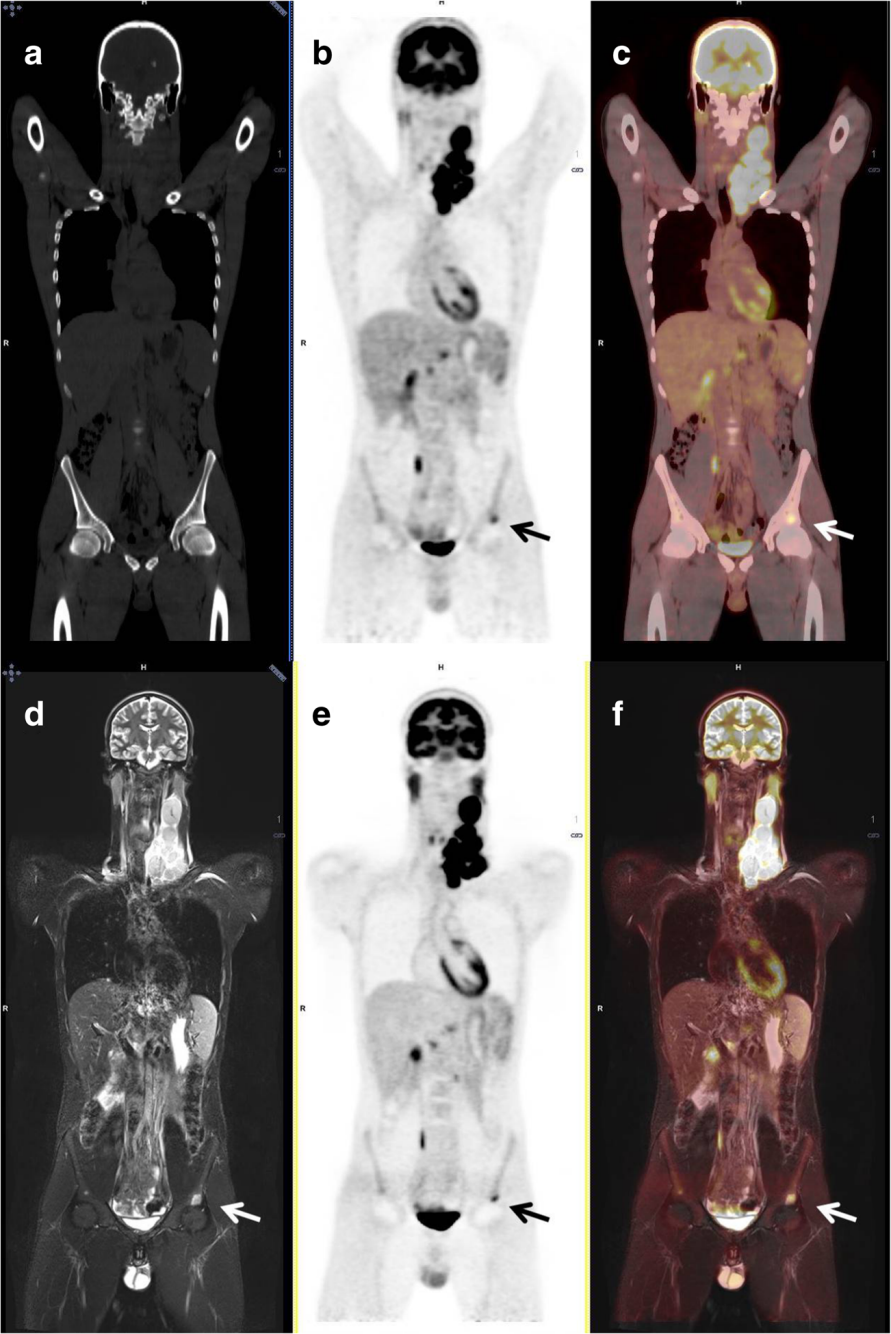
Coronal CT (a), coronal PET from PET/CT (b), fused coronal PET/CT (c), coronal STIR (d), coronal PET from PET/MRI (e), and fused coronal PET/MRI (f). FDG-avid left iliac bony lymphomatous lesion (above acetabulum; arrows) can be observed in the PET images obtained from both the PET/CT and the PET/MRI scans. However, no anatomic correlate is visible on CT, whereas it is clearly visible in the STIR image (see Supplemental Appendix Table 2 and Supplemental Appendix 3)
